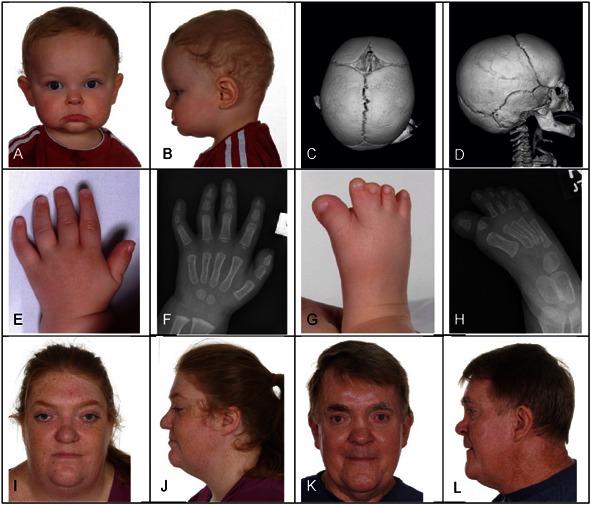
Images of British family showing craniofacial and limb features with similarity in phenotype and severity. A–H, proband: (A,B)—AP and left lateral photographs (note mild dysmorphic features and hypertelorism) (C,D)—vertex and right lateral 3D CT head (note presence of all sutures) (E,F)—photograph and X-ray of left hand (note broad radially deviated thumb, 3/4 mild incomplete syndactyly and little finger clinodactyly) (G,H)—photograph and X-ray of right foot (note broad medially deviated great toe, 2/3 complete, 4/5 incomplete syndactyly and absence of middle phalanges). I,J: Proband's mother: AP and left lateral photographs (note dysmorphic features and hypertelorsim). K,L: Proband's maternal grandfather: AP and left lateral photographs (note mildly dysmorphic features, hypertelorism, and flattened nasal bridge). [Color figure can be seen in the online version of this article, available at http://wileyonlinelibrary.com/journal/ajmga]
